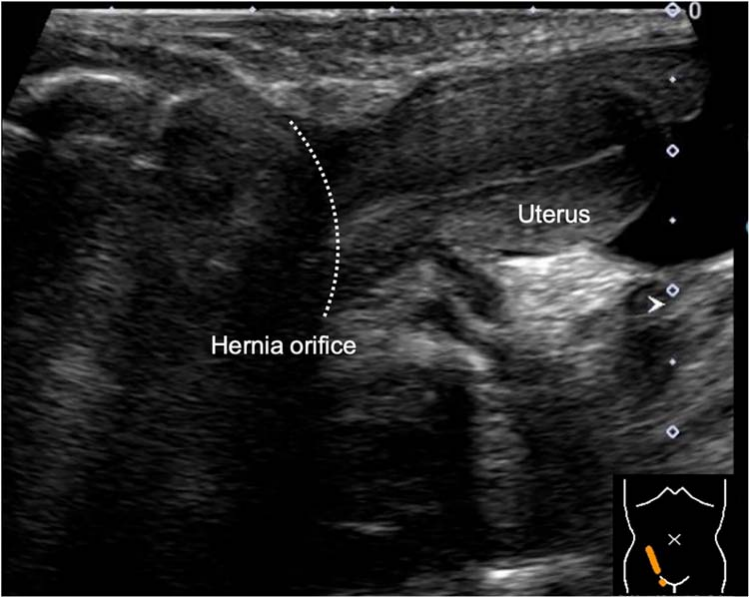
Sagittal view of the inguinal ultrasound at 3 months of age, revealing the presence of uterus protruding into the hernial sac.
